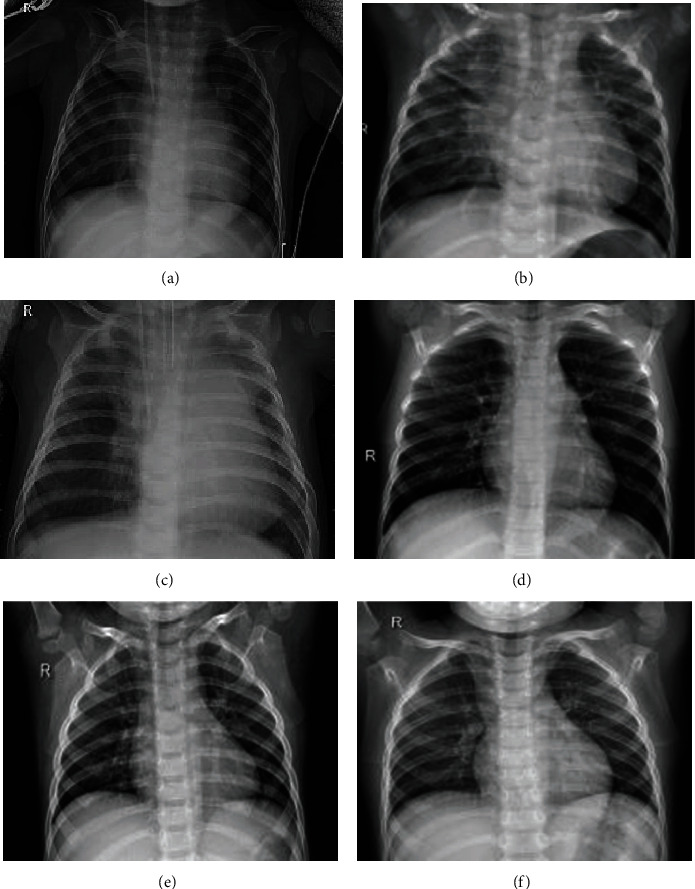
Data set format: (a–c) chest radiograph medical images of ordinary pneumonia, and (d–f) chest radiograph medical images of confirmed pneumonia. It can be roughly seen from the figure that the chest texture structure of the routine chest radiograph is more straightforward than that of the pneumonia chest radiograph.
